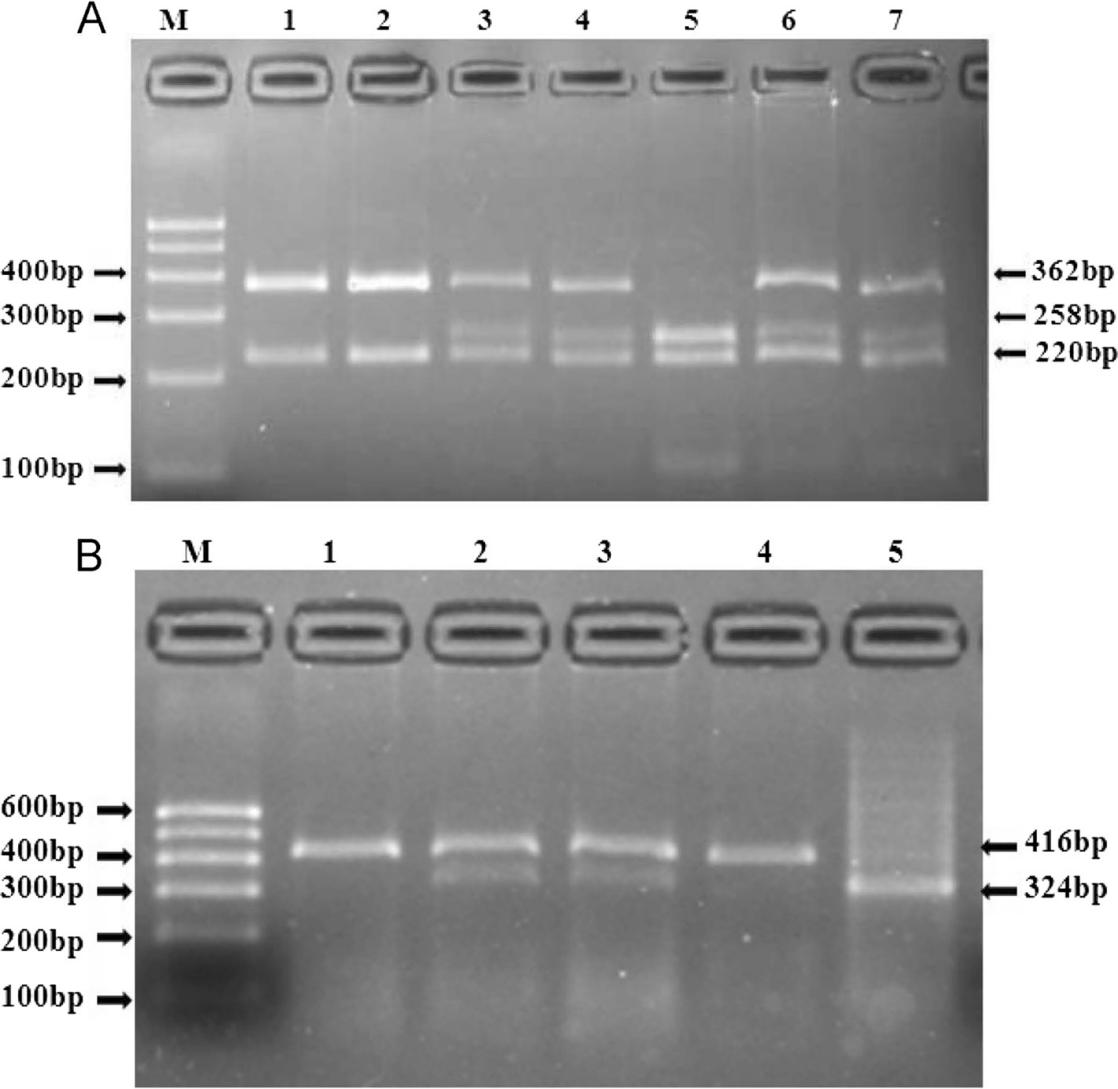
Restriction fragment length polymorphism analysis for determination of genotype. A. For SNP1, the GG genotype shows two bands of 362 bp and 220 bp (1 and 2); The AG genotype shows three bands of 362 bp, 258 bp and 220 bp (3, 4, 6 and 7), The AA genotype shows three bands of 258 bp, 220 bp and 104bp (5). B. For SNP2, the TT genotype shows one band of 416 bp (1 and 4); The CT genotype shows two bands of 416 bp and 324 bp (2 and 3); The CC genotype shows one band of 324 bp (5).
